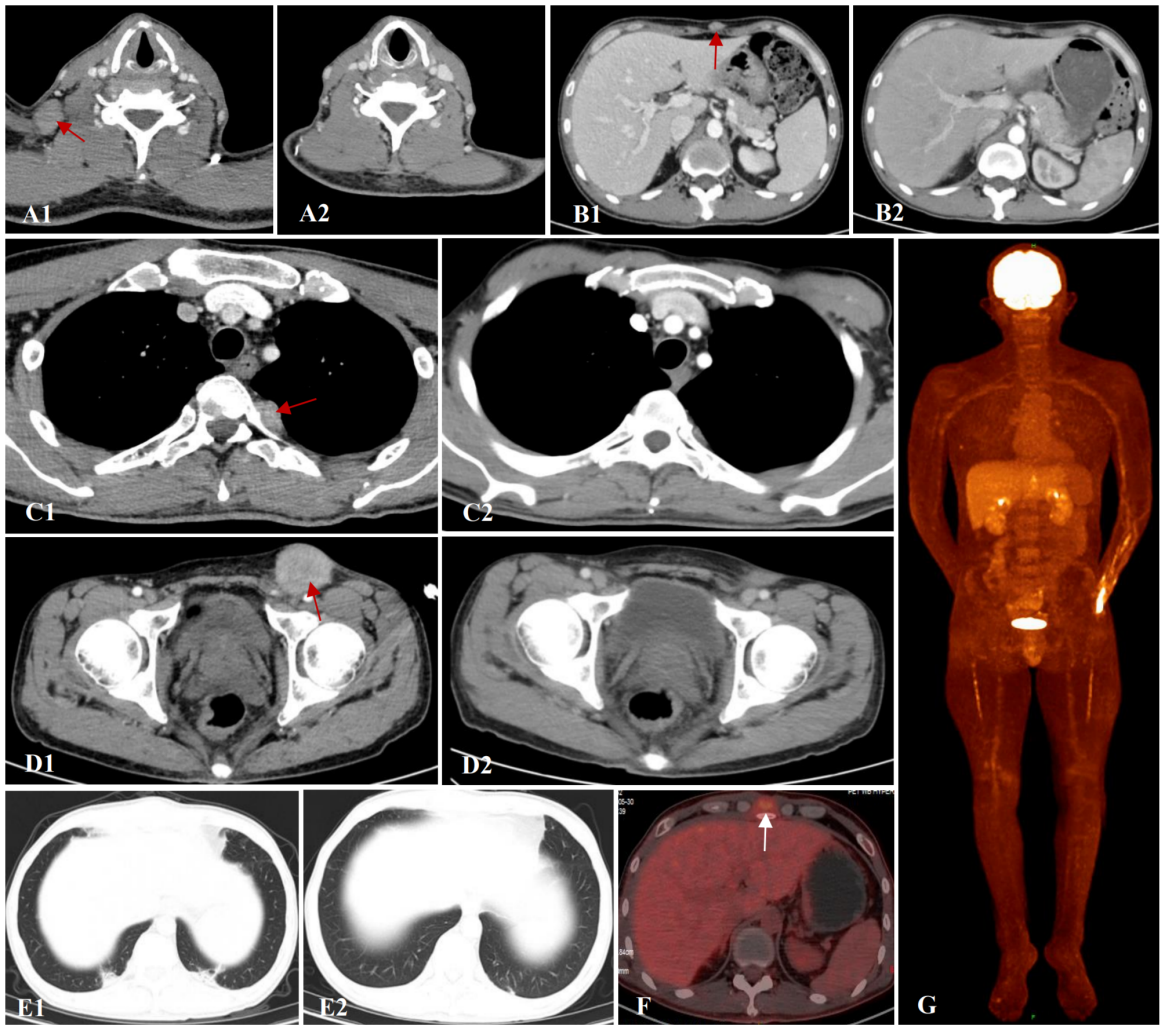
Contrast-enhanced CT of the neck, chest and abdomen. (A1, A2) Axillary lymph nodes before and after VCA treatment. (B1, B2) Soft tissue mass around the subxiphoid before and after VCA treatment. (C1, C2) Paravertebral soft tissue image before and after VCA treatment. (D1, D2) Soft tissue mass in the inguinal region before and after VCA treatment. (E1, E2) Pulmonary infection before and after antifungal therapy. (F, G) PET-CT revealed active ^18^F-FDG metabolism in the superficial fascia and xiphoid process of the right chest wall after VCA treatment.
